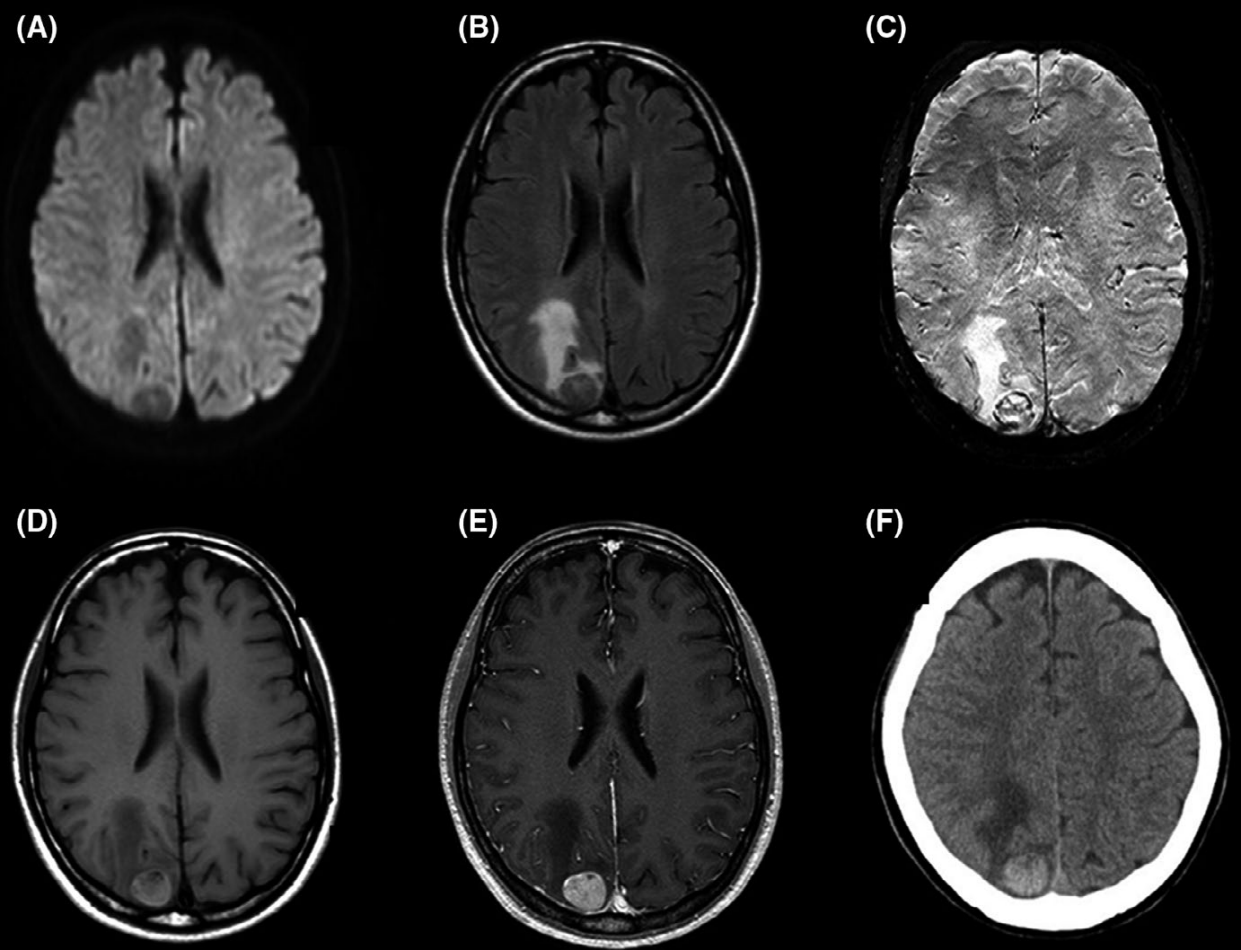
Brain magnetic resonance imaging (MRI) and computed tomography (CT) on admission. Brain MRI showed a mass lesion 2 cm in diameter at the cortico‐medullary junction in the right occipital lobe. It was hypointense on diffusion‐weighted imaging. (A) Fluid‐attenuated inversion recovery imaging revealed coexistence of hypo‐ and hyperintense areas surrounded by hyperintense lesion in white matter, suggesting edema. (B) T2*‐weighted imaging showed hypointense area at the edge. (C) The lesion was hyperintense on T1‐weighted imaging (D) and later turned out to be hyperintense on gadolinium contrast–enhanced imaging. (E) The lesion was hyperdense on CT. (F)
